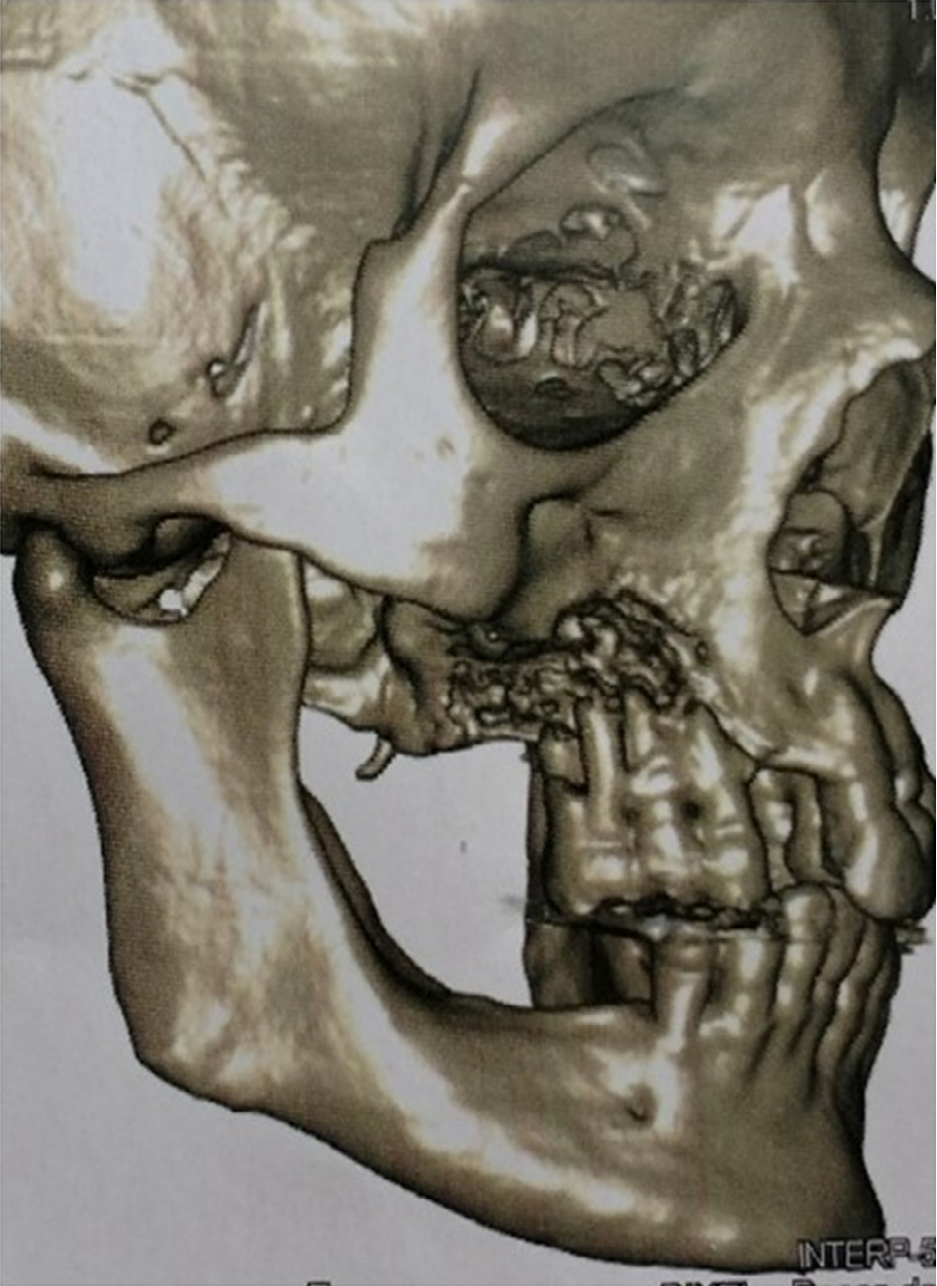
3D CT reconstruction prior to the first biopsy.
